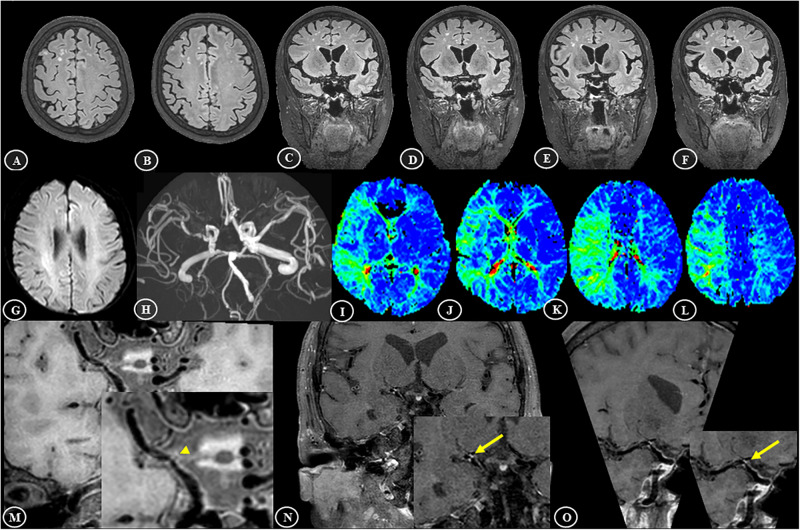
Representative brain MR findings of a 74-year-old female patient with white matter hyperintensities and intracranial atherosclerosis. The patient was admitted with dizziness for 1 month. (A–F) On the 3D-FLAIR images, asymmetrically distributed white matter hyperintensities, mainly in the right deep white matter area, are shown. (G) Diffusion-weighted imaging (DWI) showed a normal appearance. (H) 3D-TOF MRA showed significant stenosis in the M1 segment of right middle cerebral artery (MCA). (I–L) According to the Alberta Stroke Program Early CT score (ASPECTS), the TTP maps showed a large area of hypoperfusion, including M1–6 and the insular ribbon, and the TTP-ASPECTS was 3. (M–O) T_1_-VISTA image showed an isointense plaque in the right MCA (arrowhead), and the contrast-enhanced T_1_-VISTA image showed strong enhancement of the plaque (grade 2) (arrows).
